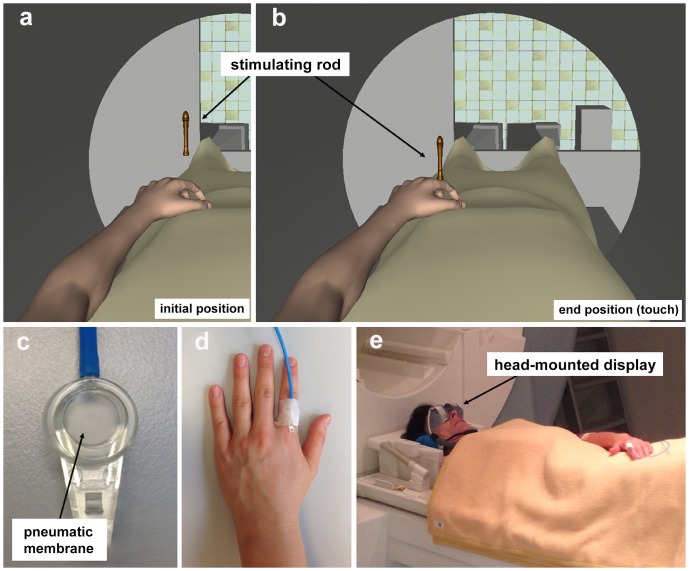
Setup of the virtual hand illusion. a) participant's view of the virtual reality environment, including the virtual limb and the stimulating rod at its starting position, b) the same view and rod at its end position touching the virtual finger, c) close-up picture of the pneumatic tactor, d) pneumatic tactor, attached to the participants' left index finger, e) participant lying on the table connected with the magnetic resonance imaging scanner. The participant has given written informed consent, as outlined in the PLOS consent form, to publication of her photograph.
